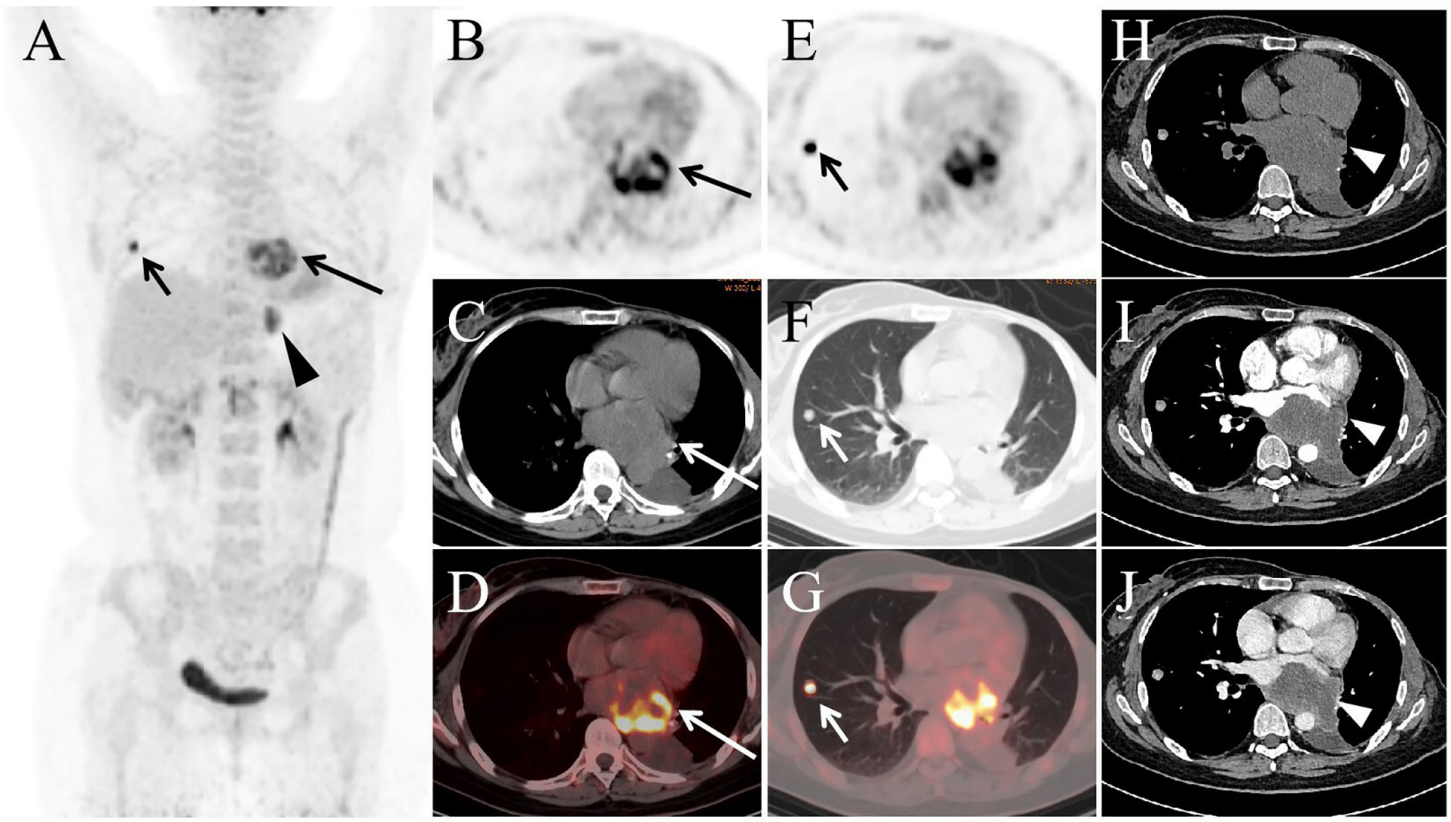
The MIP image (A) revealed increased FDG activity in lesions located in the heart (long arrow), right lung (short arrow), and left pleura (arrowhead). Transaxial images (B: PET, C: CT, D: PET/CT) displayed a large soft tissue mass in the left atrial region, exhibiting significantly elevated FDG activity with an SUVmax of 9.5. Additionally, a nodule was observed in the right upper lobe (E-G), showing abnormal FDG uptake with an SUVmax of 10.1. Contrast-enhanced CT (H-J) identified mild enhancement of the mass in the left atrium (arrowhead).
